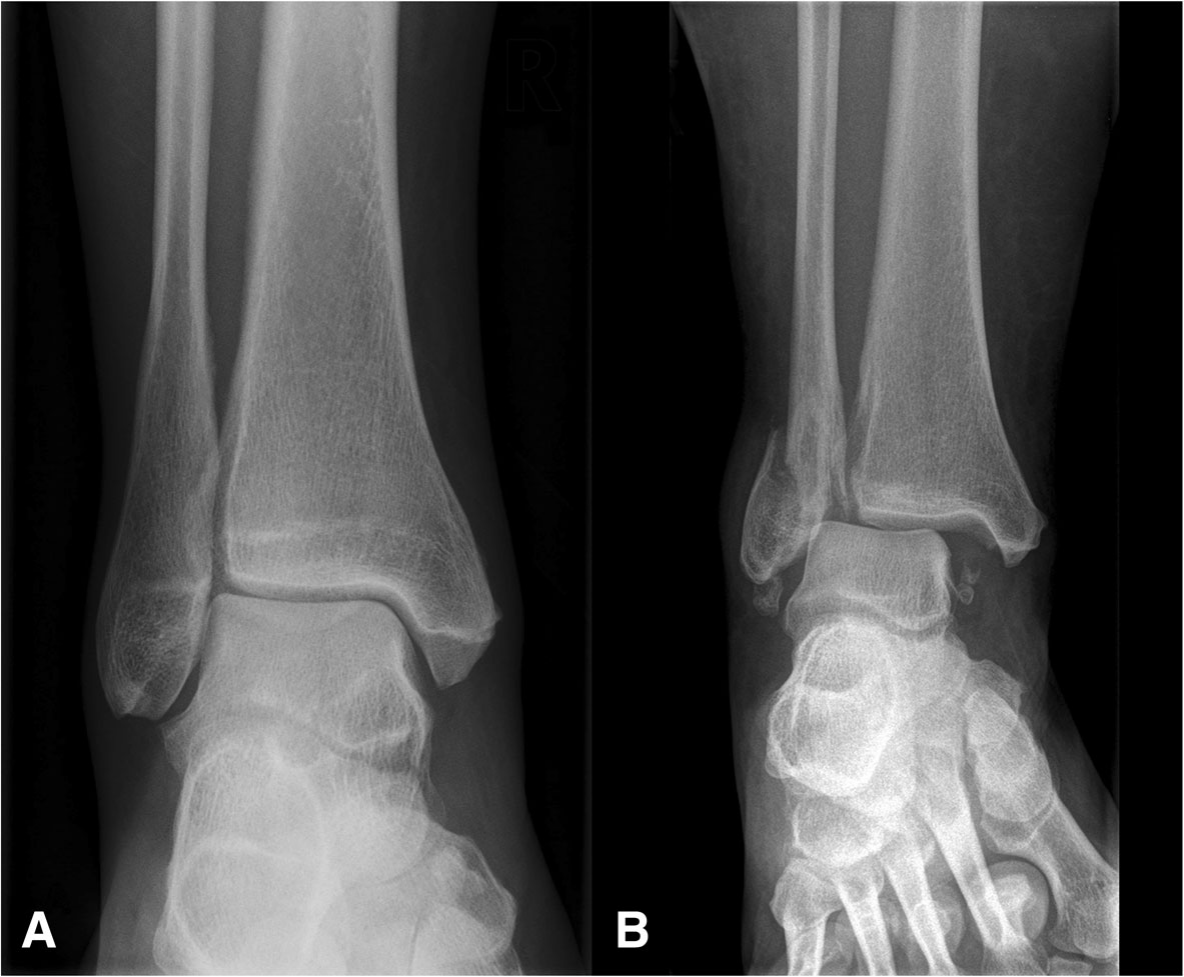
Examples of radiographs used in the study (a no fracture, b fracture present)
